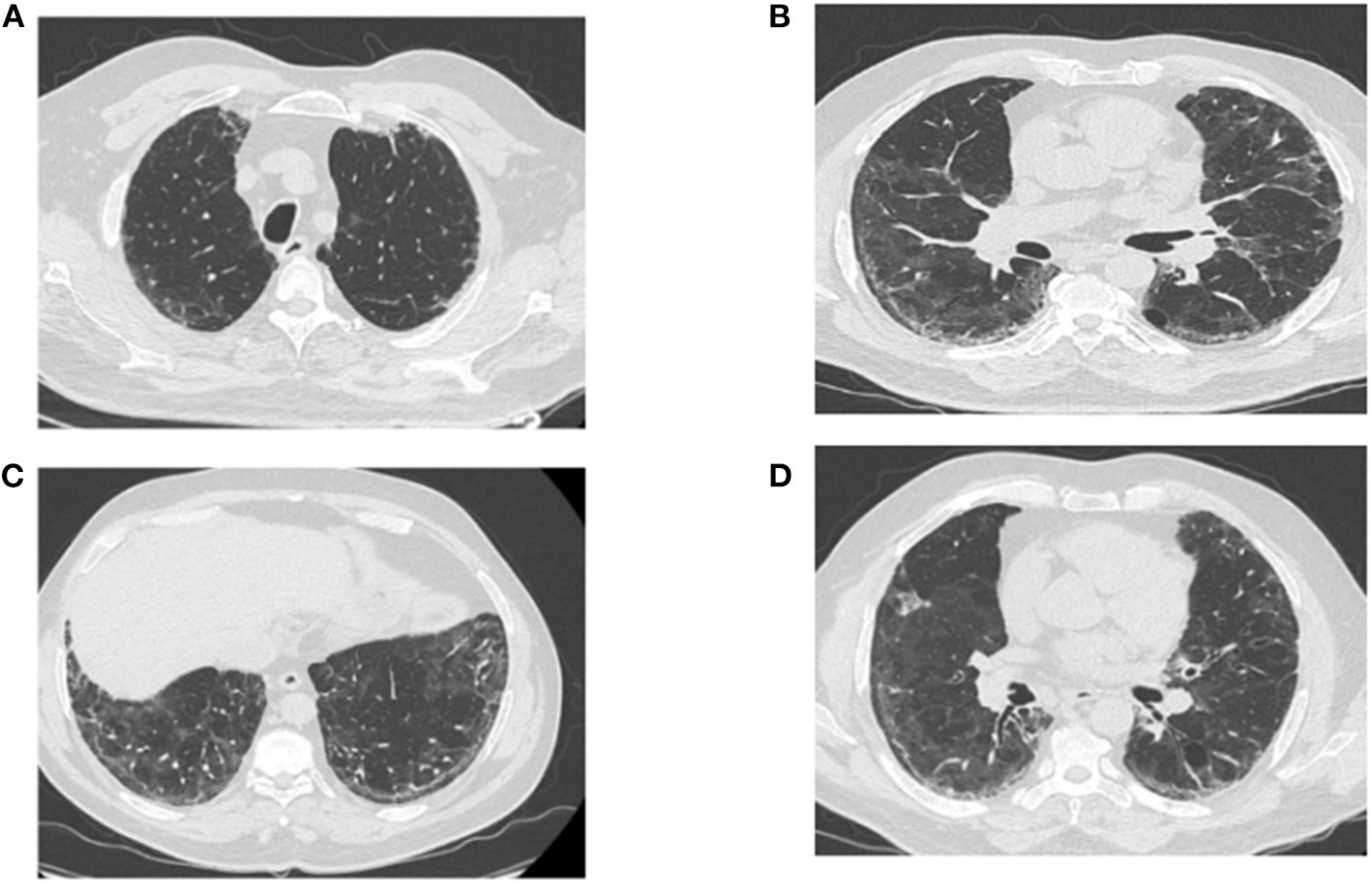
Post-corticotherapy chest CT scan (third chest CT scan). The chest CT scan performed after 3 months of corticotherapy of post-COVID-19 symptoms reveals the regression of ground-glass lesions and bronchiectasis, and the persistence of paracicatricial emphysema (A–C). (B–D) Show the disappearance of the cavity image of the right middle lobe, the regression of retraction signs, and the persistence of few reticulation lesions.
